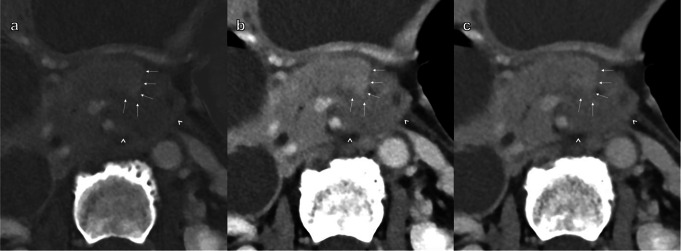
Axial contrast-enhanced abdominal CT scan in portal venous phase. Image a is MD-iodine, image b is VMI at 55 keV, and image c is VMI at 70 keV. The images are of a 56-year-old female patient with a PDAC in the body of the pancreas. The white arrows indicate the border between the tumor and normal pancreatic parenchyma. The arrowheads indicate the border between the tumor and the retroperitoneal fat
